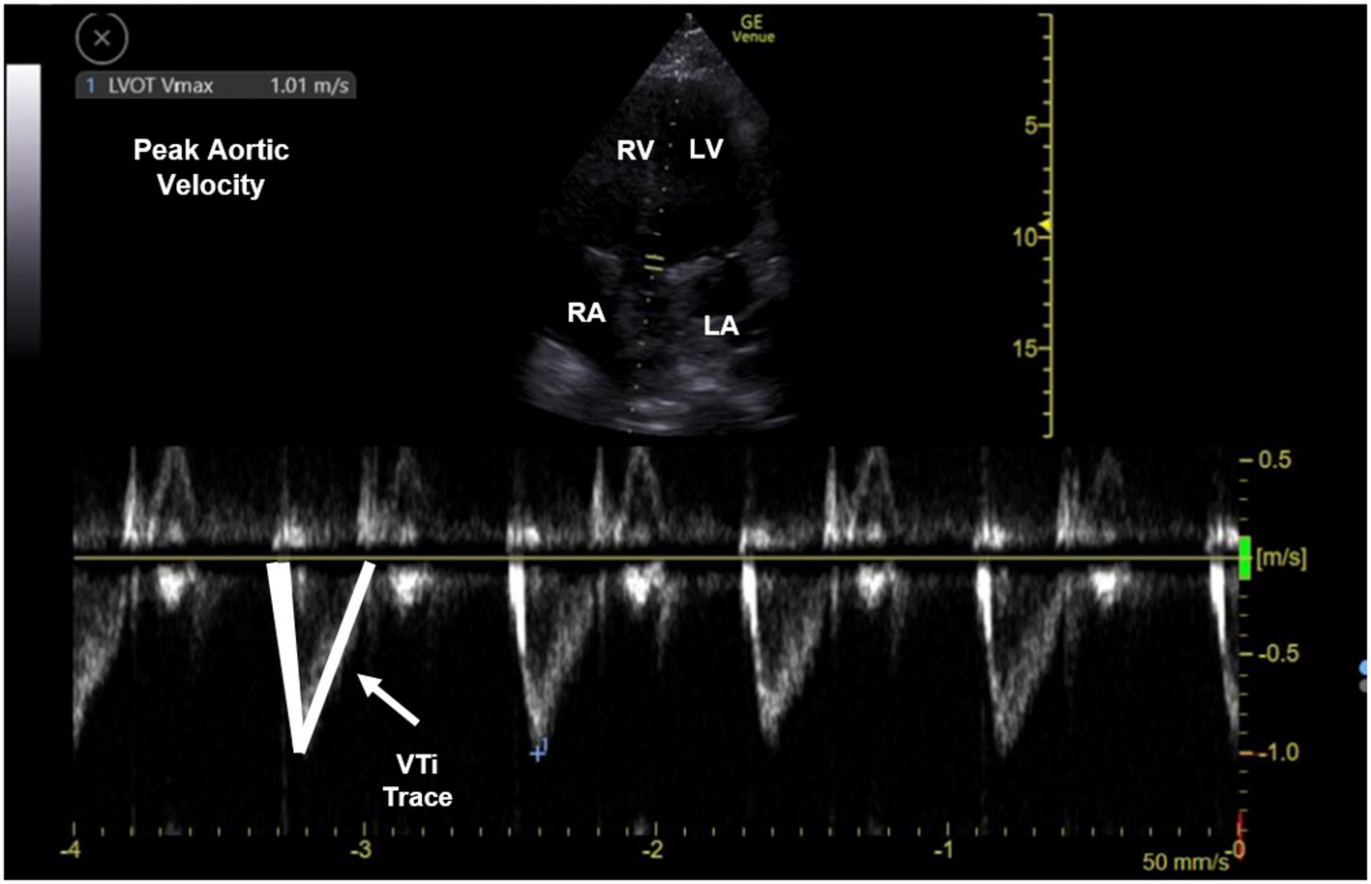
Pulse doppler waveform measuring peak aortic velocity and velocity time integral (VTi). The upper half of the image displays an apical 5 chamber view with pulse doppler gate at the aortic outflow tract. The lower half of the image displays doppler waveform (m/sec). The waveform is traced to estimate VTi and measure peak aortic velocity. RV, right ventricle; LV, left ventricle; RA, right atrium; LA, left atrium; VTi, velocity time integral.
